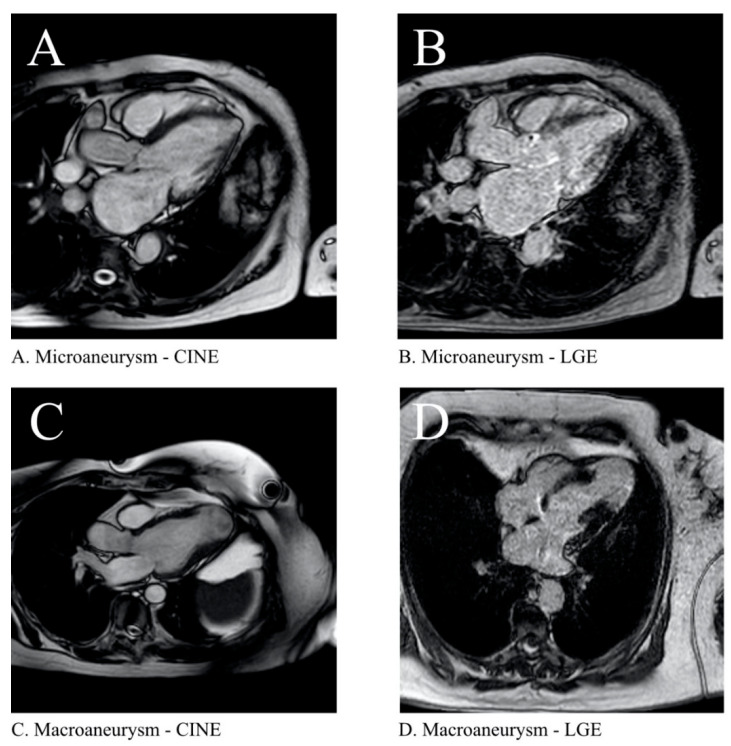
Cardiac Magnetic Resonance Imaging in Chagas disease. (CINE: Steady state free precession sequence. LGE: Late gadolinium enhancement).
